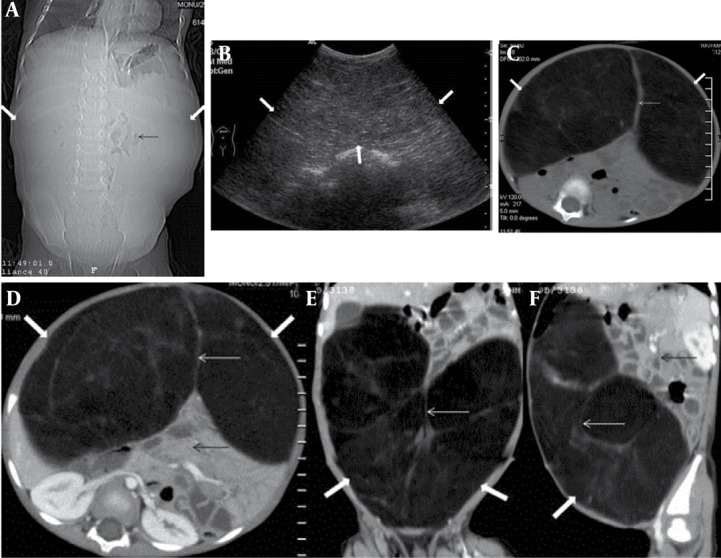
A 2.5-Year-Old Boy Presenting With Complaints of Gradual Abdominal Distention, Intermittent Abdominal Pain and Diarrhea. A, Scannogram of the abdomen shows gross abdominal distension (thick white arrow) and centrally positioned bowel loops (thin black arrow). B, Abdominal ultrasound in the same patient reveals a huge homogeneous echogenic mass (thick white arrow) occupying almost the entire abdomen. C, (Plain) and D, E, F (Contrast-enhanced axial/coronal/sagittal) abdominal CT images of the same patient reveal a well-marginated non-enhancing intraperitoneal encapsulated mass (thick white arrow) of fat density (-70 to -140 HU) with few fibrous septae (thin white arrow) traversing the mass. Bowel loops and retroperitoneal structures are displaced posteriorly (thin black arrow). No inflammatory reaction or infiltration of surrounding tissues is noted.
